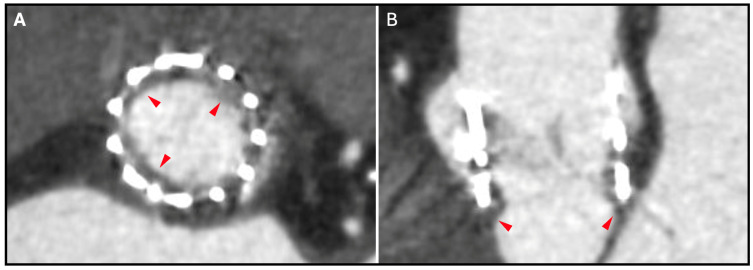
Example of THV pannus. Example of THV pannus (A) short axis and (B) double oblique transverse plane showing a circular circumferential hypodense tissue (red arrowheads) on the ventricular side at the inflow of the THV suggestive of pannus formation. CT: computed tomography; THV: transcatheter heart valve. CT showing a circular circumferential hypodense tissue (red arrowheads) on the ventricular side at the inflow of the THV (black arrows) suggestive of pannus formation. CT: computed tomography; THV: transcatheter heart valve.
